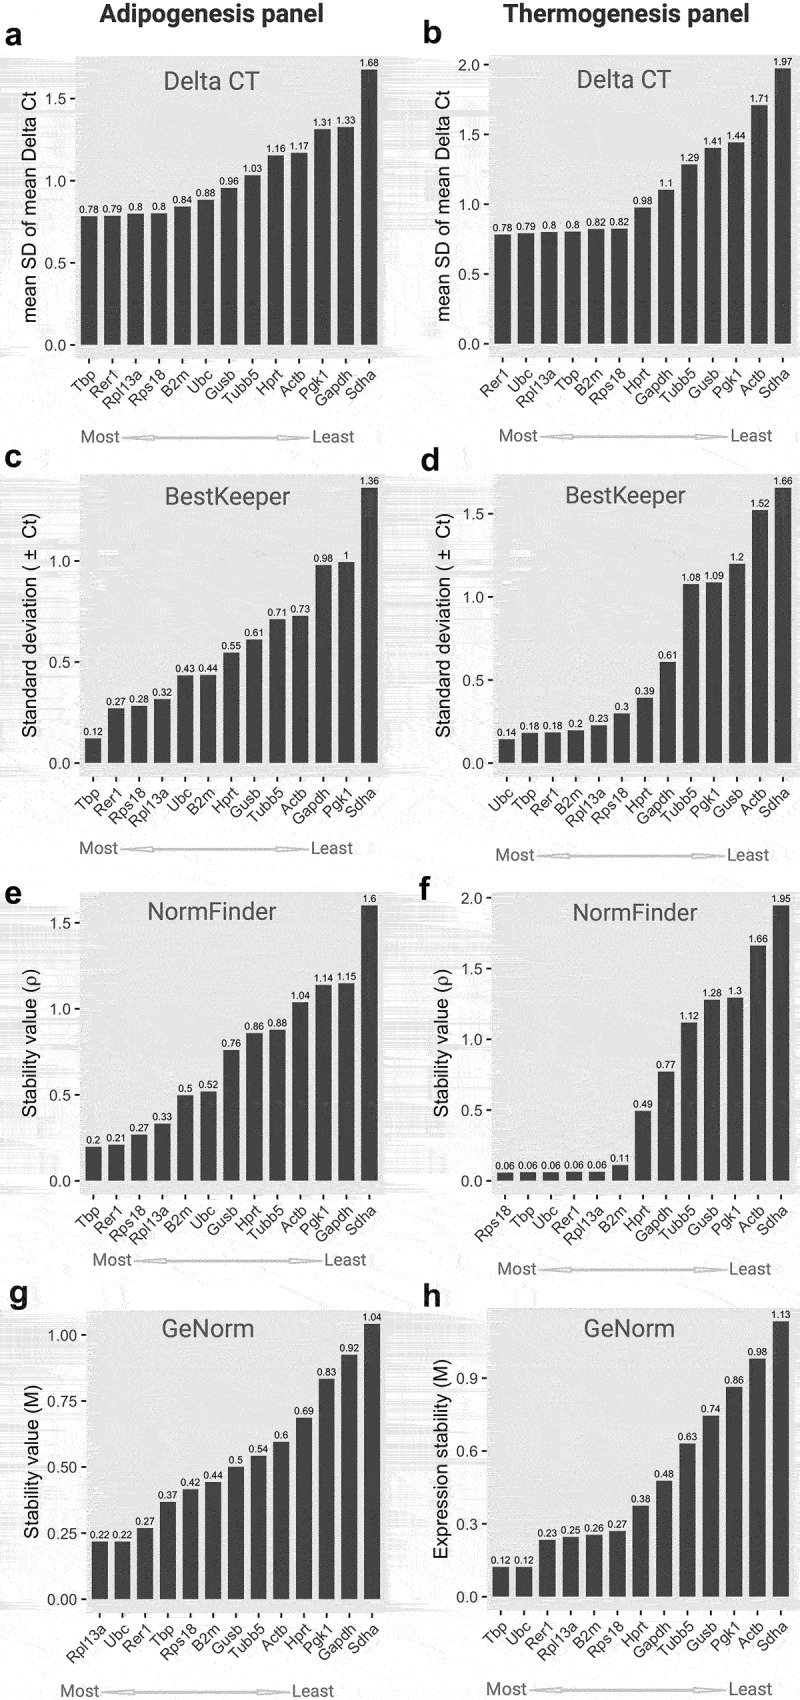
Stability scores and ranking of 13 candidate housekeeping genes in the adipogenesis and thermogenesis panel. (a,b) Stability scores from *Delta CT* algorithms; (c,d) Stability scores from *BestKeeper* algorithms; (e,f) Stability scores from *NormFinder* algorithms; (g,h) Stability scores from *GeNorm* algorithms at an adipogenesis (left) or thermogenesis (right) panel, respectively. For the adipogenesis panel, cells were collected at day 0, day 2, day 4, and day 8 during adipocyte differentiation. For the thermogenesis panel, cells at day 0 and day 8 were treated with norepinephrine (1 *µ*M) or DMSO and incubated for 24 hours. All experiments were performed at three biological replications. The mRNA expression of reference genes underwent examination through RT-qPCR and the RefFinder tool was utilized for calculating stability scores. Original Ct values, including biological replications, from all time points in adipogenesis condition or all treatments in thermogenesis were inputted into the RefFinder tool for analysis. The stability score of individual candidate gene, determined by each algorithm, was displayed as bar-graphs. Lower (left) and higher (right) scores indicate more and less stable genes, respectively.
